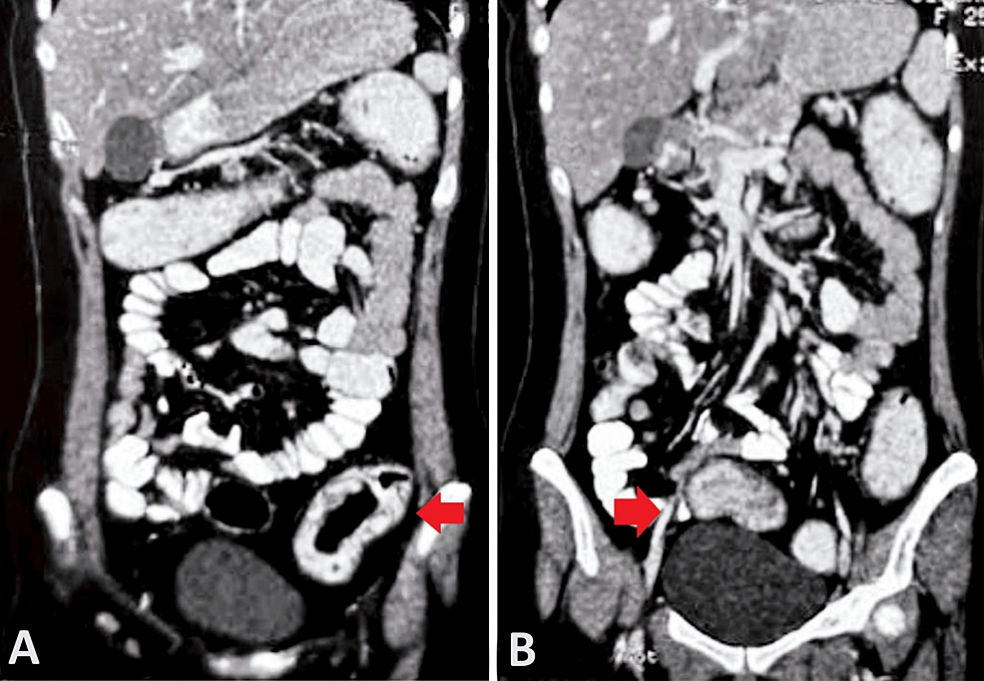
Contrast-enhanced computed tomography scan of abdomen and pelvis coronal sections (A) and (B) showing heterogeneously enhancing diffuse edematous wall thickening involving the rectosigmoid colon (red arrows)
